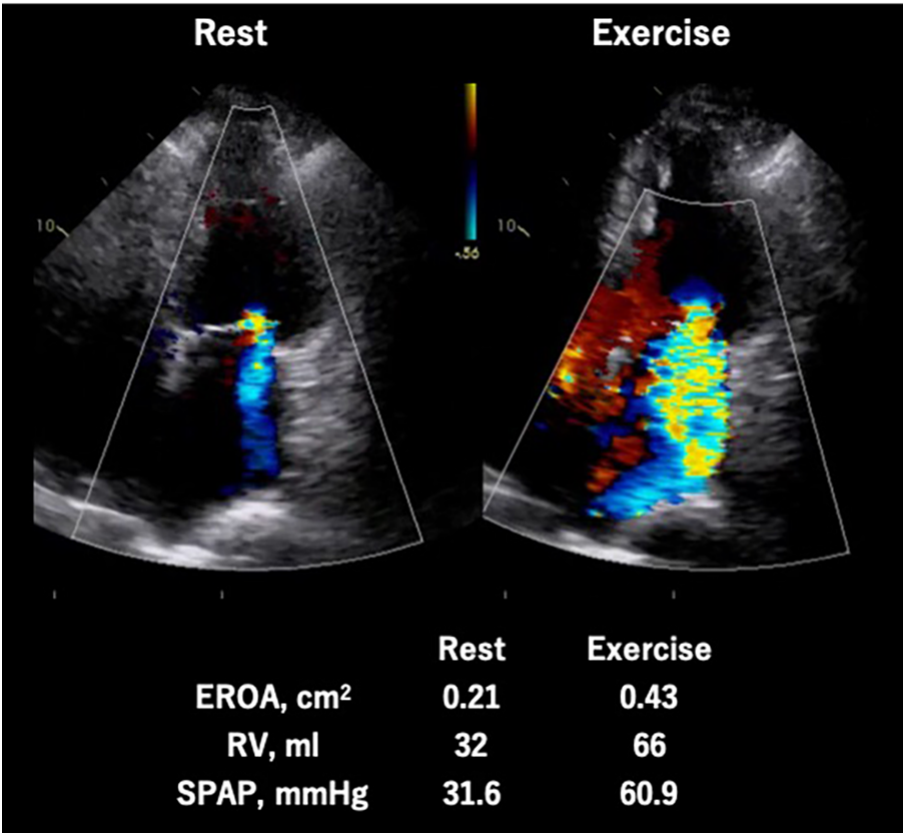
Exercise stress echocardiography for mitral stenosis. Eighty-four-year-old woman, a case of calcified severe MS confirmed by ESE. During exercise, MMPG increased from 5.7 mmHg to 24.9 mmHg and significant Ex-PH was observed. ESE, exercise stress echocardiography; MMPG, mean mitral pressure gradient; MS. mitral; stenosis; SPAP, systolic pulmonary arterial pressure.
